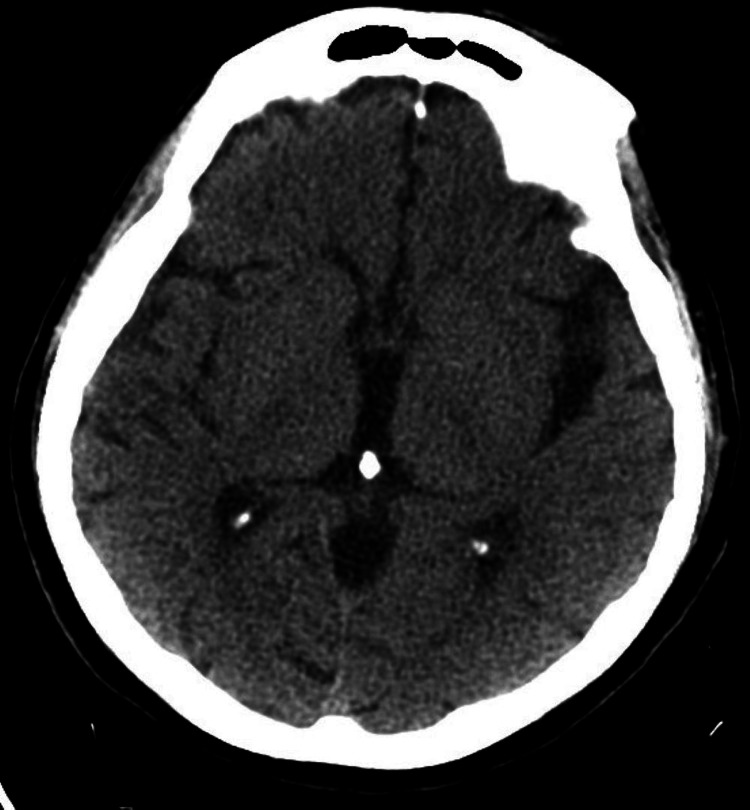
CT brain showing no evidence of acute hemorrhage or infarct, no midline shift, no mass effect, no extra-axial fluid collection and normal osseous structures
